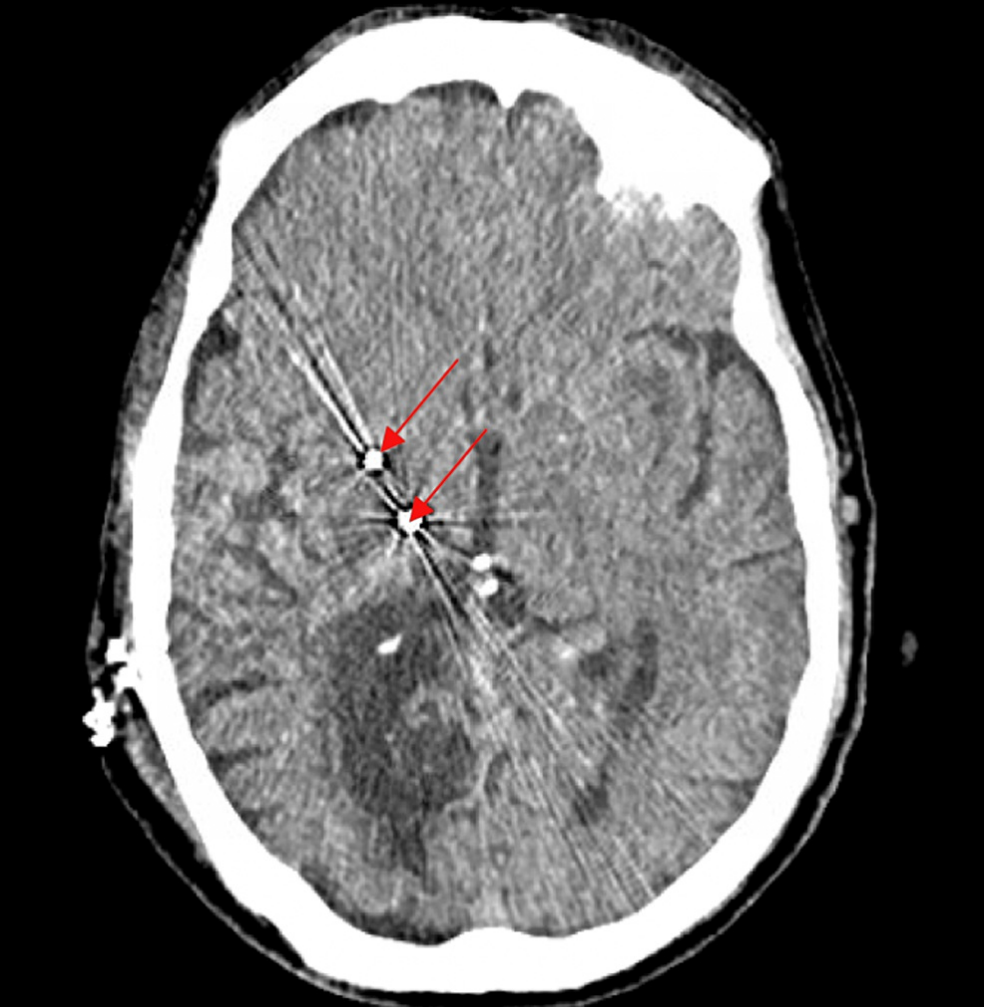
Non-contrast CT of the head showing the presence of right deep brain stimulator placement with electrode tips (red arrows) about the right globus pallidus internus and ventral intermediate nucleus of the thalamus
